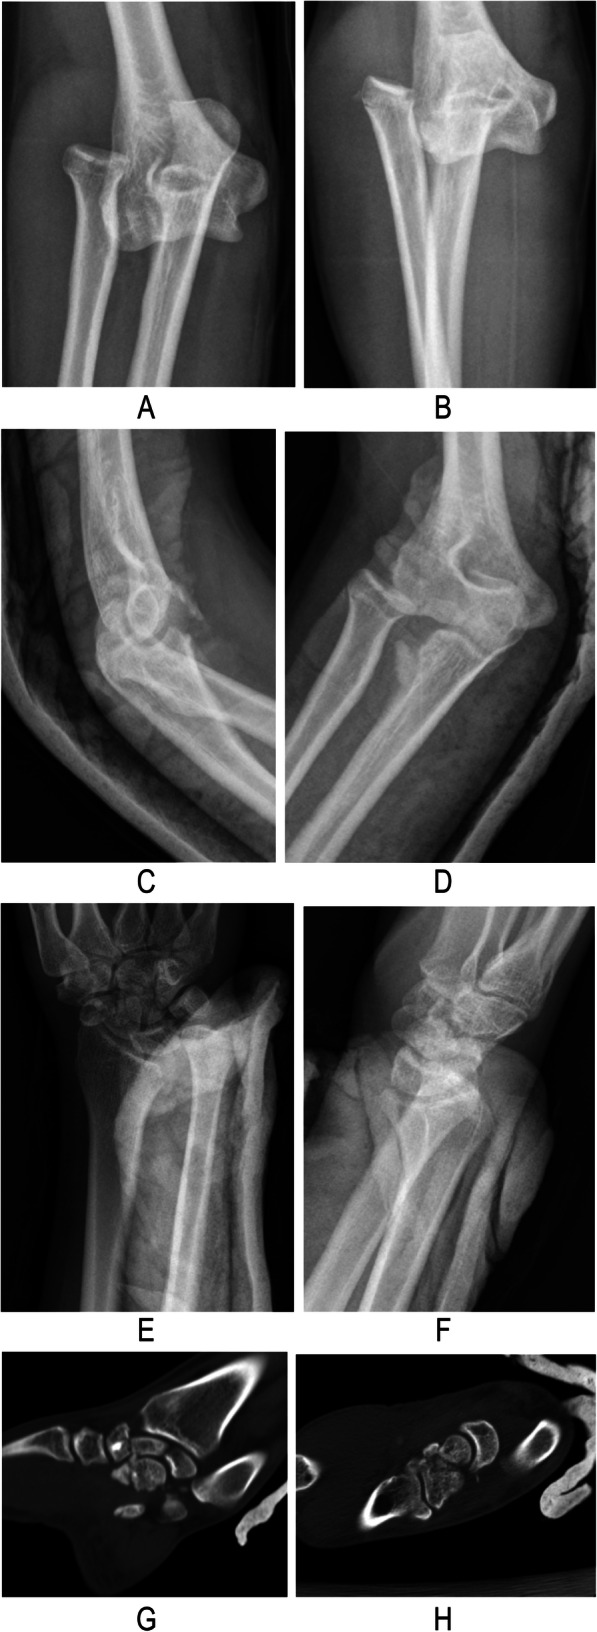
First radiographs, a anteroposterior view, b oblique view; c-d elbow joint after reduction; e-f wrist joint; g-h wrist joint CT
